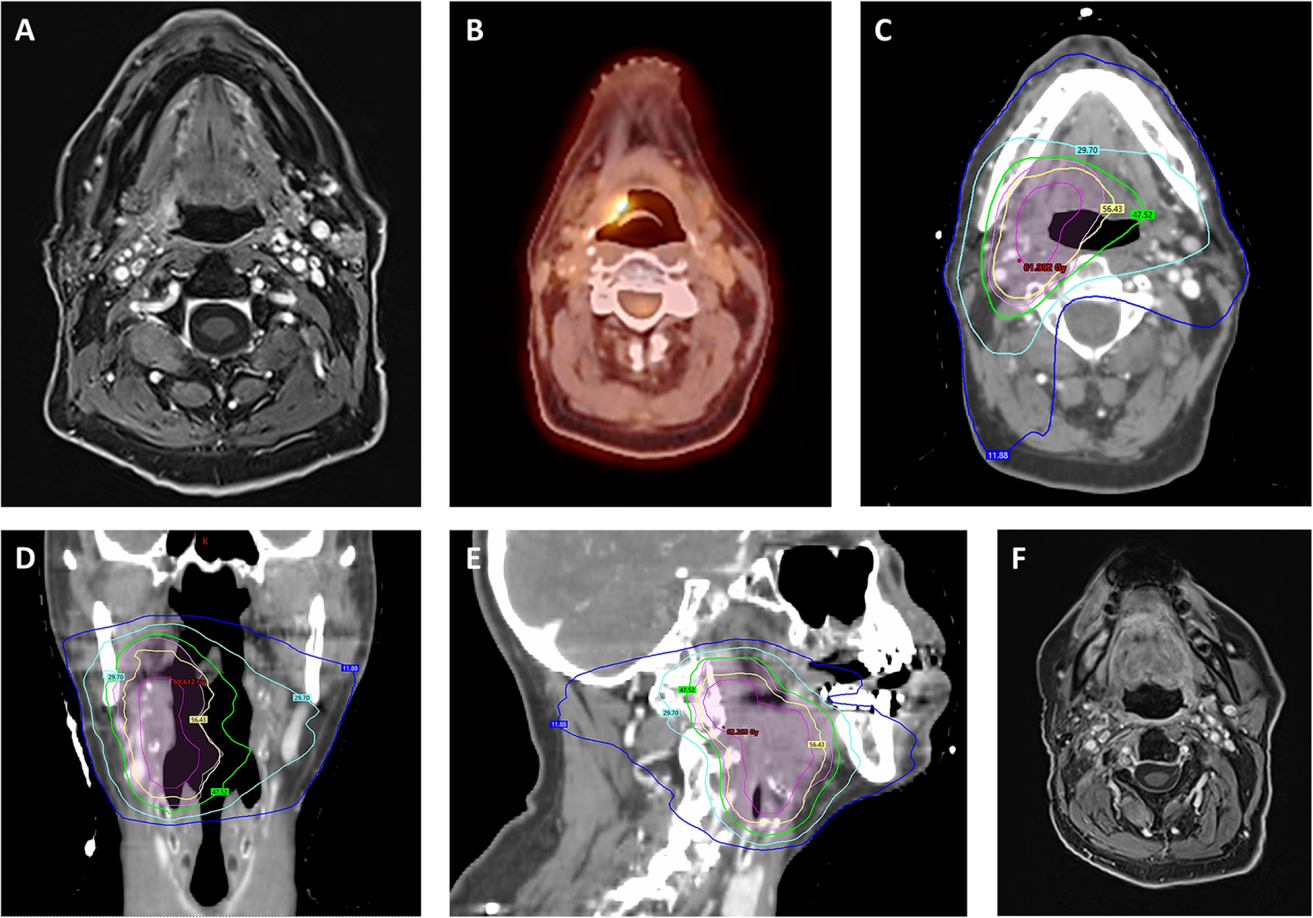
Re-irradiation for a recurrent oropharynx carcinoma in a 79-year-old male patient. The patient received chemoradiotherapy with 70 Gy for a HPV-positive cT4 cN2c cM0 oropharyngeal carcinoma (7th Edition of the UICC TNM classification) between March and May 2010. In July 2017, the patient developed a recurrent HPV-positive rcT2 rcN1 cM0 oropharyngeal carcinoma which was treated by re-irradiation with 52.2 Gy between September and October 2017. Initially, a total re-irradiation dose of 59.4 Gy was planned, but the treatment was discontinued after worsening of the patient’s general condition. Pretherapeutic MRI imaging (a) in July 2017 and FDG-PET-CT imaging (b) in August 2017 showing the recurrent rcT2 rcN1 cM0 oropharyngeal carcinoma on the right side. Based on the recommendations of the multidisciplinary tumor board, re-irradiation using IMRT was performed. Dose distribution of the IMRT plan is shown in an axial (c), coronal (d) and sagittal (e) scan image. PTV1 (pale pink) receiving 50.4 Gy in 28 fractions and PTV2 (pink) receiving 9 Gy in 5 fractions as sequential boost are shown as well as the lines for the 95%-isodose (yellow), 80%-isodose (green), 60%-isodose (cyan) and 20%-isodose (blue). The last MRI in November 2019 (f) showed no signs of recurrence
